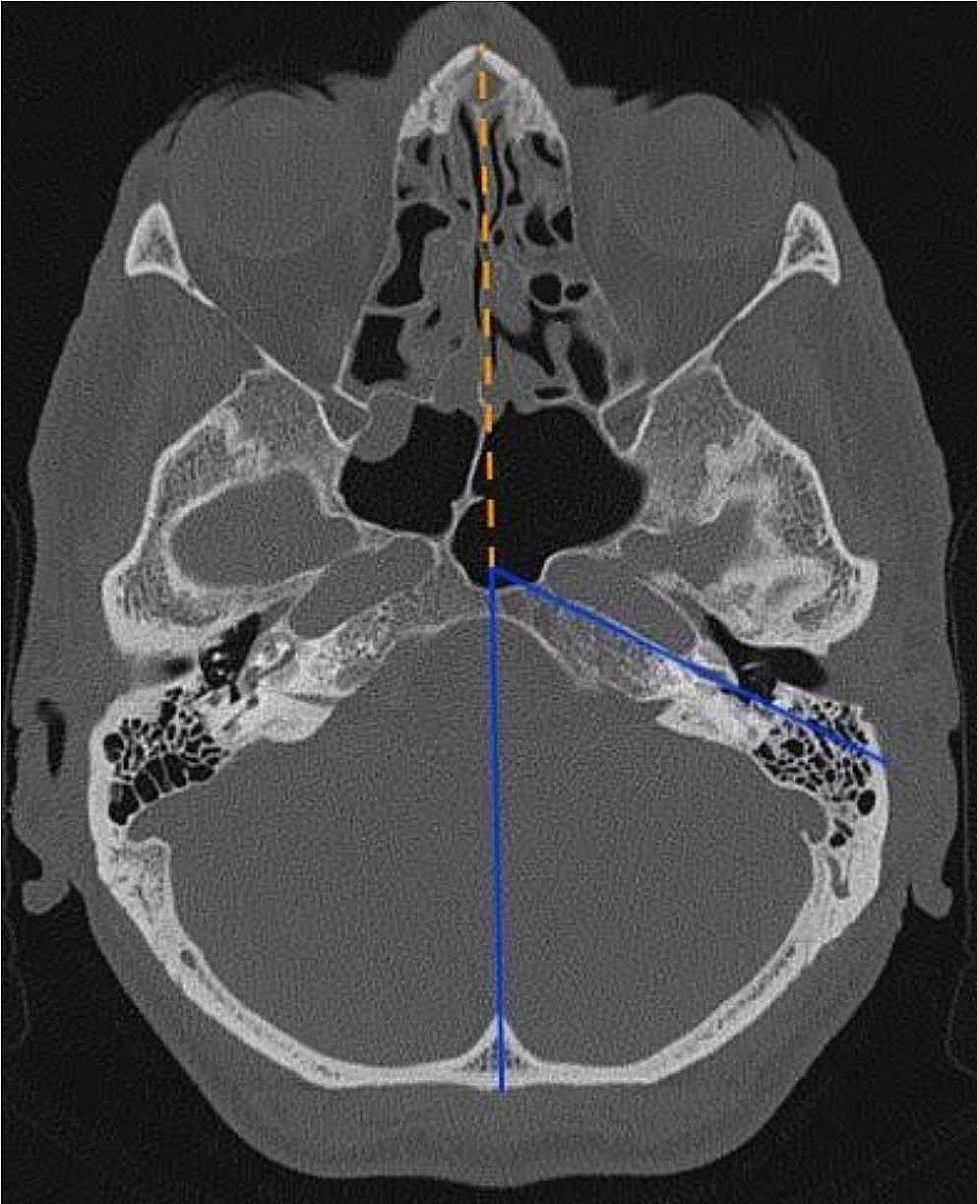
Computed tomography of the ear, both ears can be seen, axial plane. The measurement described by Xie et al. [21] is indicated by the angle between the two lower (solid blue) lines. Code: Xie_Angle
